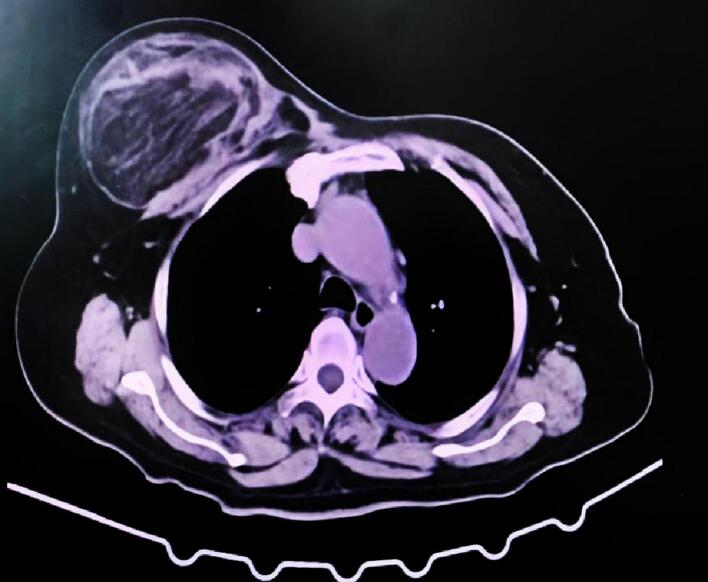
Chest CT shows a mixed density mass shadow in the right breast, with unclear demarcation from adjacent chest wall muscles.
